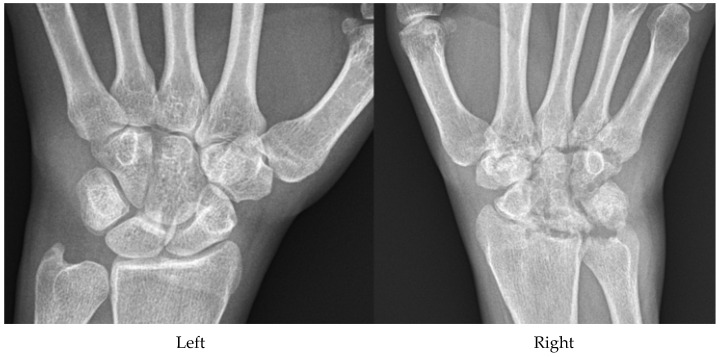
37-year-old female with septic arthritis of the right wrist. Patient presented with pain and swelling in the right wrist for a few weeks along with fever and chills. Radiographs show erosive changes in the right wrist with soft-tissue swelling. No other joints are involved. Septic arthritis is the diagnosis of exclusion in all patients presenting with monoarthritis and should not be overlooked.
